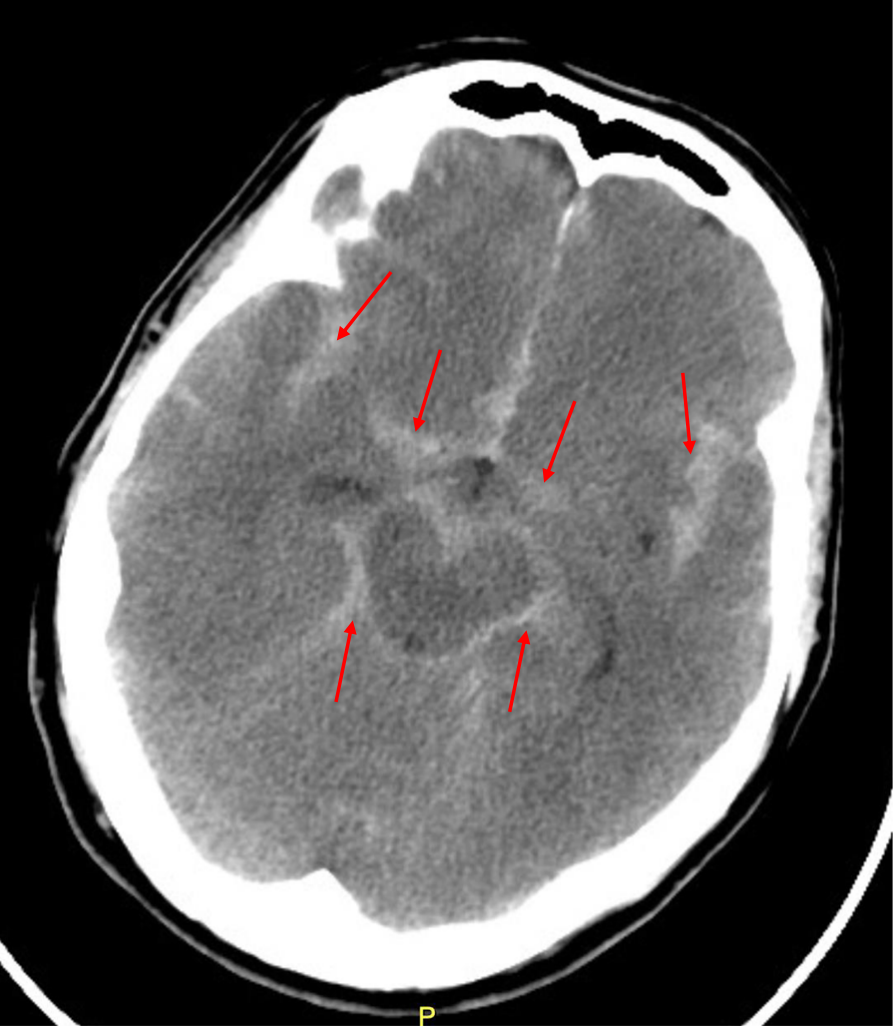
Subarachnoid hemorrhage on CT head
